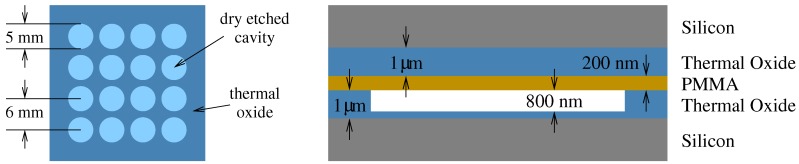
Top (left) and cross-sectional (right) views of the proposed Capacity Micromachined Ultrasonic Transducer (CMUT), showing device dimensions.
